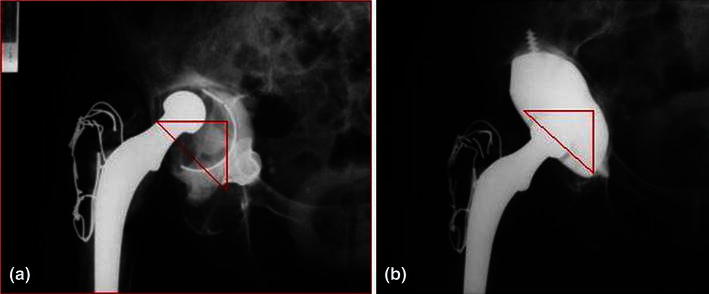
Radiographs showing acetabular reconstruction using a BOFOR cup. a Preoperative radiograph showing the center of rotation outside Ranawat’s triangle. b Postoperative radiograph showing the center of rotation inside Ranawat’s triangle
